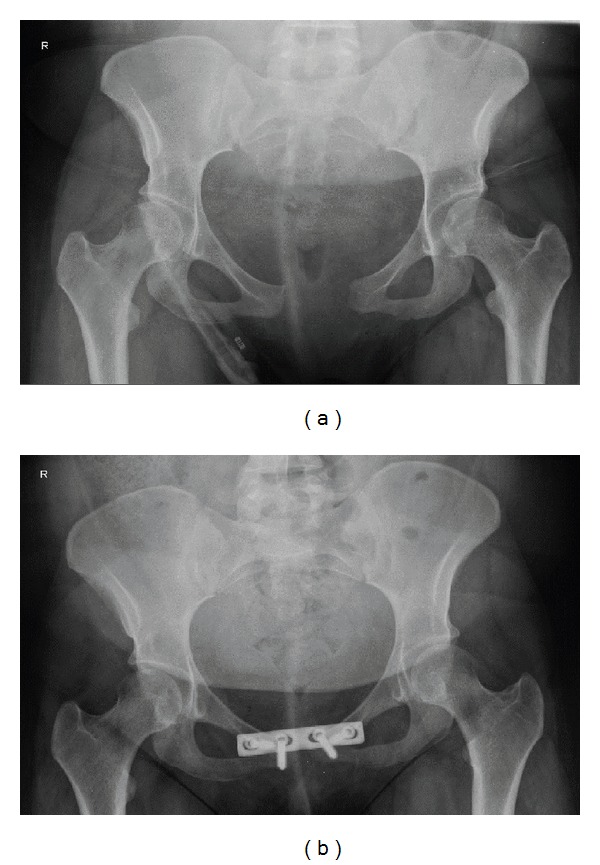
X-ray of the pelvis with a pubic symphysis separation is shown in (a). X-ray of the pelvis after surgical fixation of the symphysis is shown in (b).
